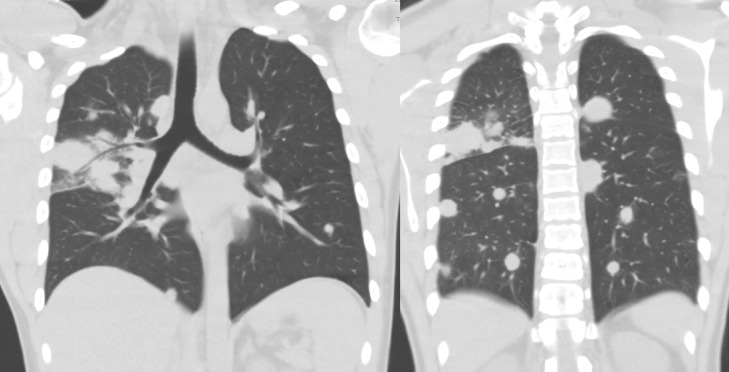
CT of the chest coronal images, showed numerous (more than 30) bilateral pulmonary nodules/masses, ranging from 20-60 mm in diameter with associated peripheral halo sign. No pleural effusion or pneumothorax and patent central airway. Findings were favored to represent vasculitis.
